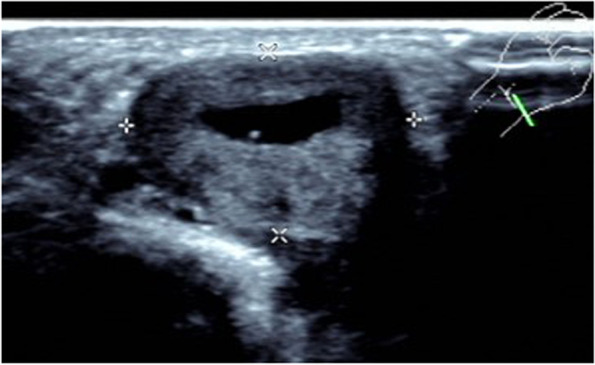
A 28-year-old man with a painless mass on the extensor surface of the wrist. Sonography shows an inhomogeneous hypoechoic nodule with an anechoic cystic area inside
